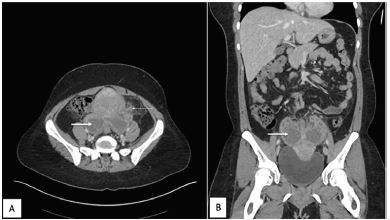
Computed tomography of abdomen and pelvis (A) axial view and (B) coronal view. Thick arrow: Bilateral adnexal multilocular septate cystic masses with enhancing septa and loss of normal ovarian parenchyma. Thin arrow: surrounding peritubal fat stranding.
